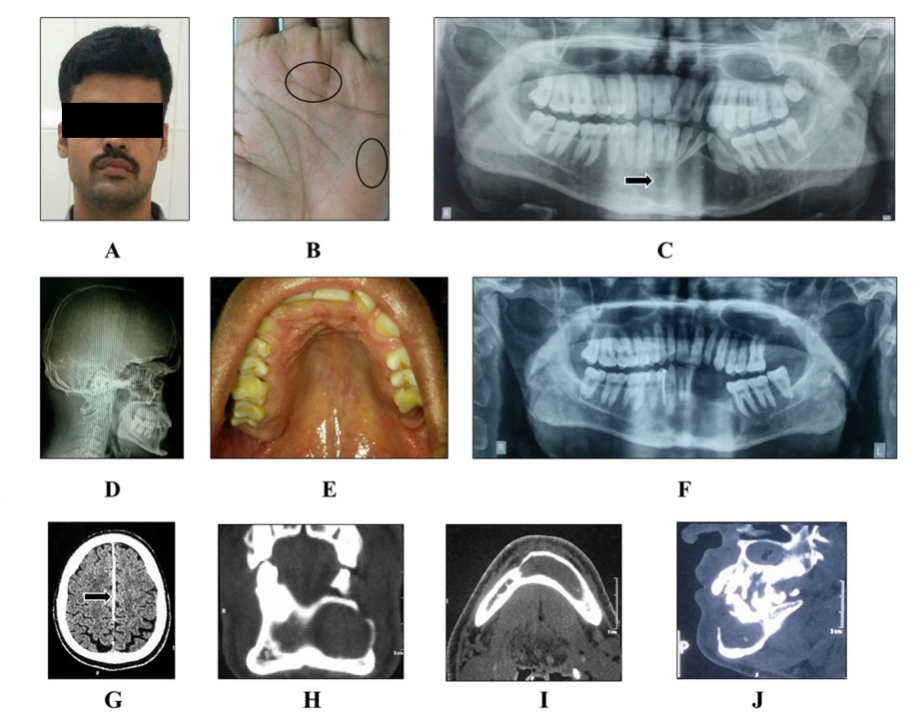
(A) Extraoral profile; (B) Palmar pits; (C) Panoramic radiograph; (D) Lateral cephalogram; (E) Intraoral examination, (F) Postoperative panoramic radiograph; (G, H, I, J) CT scan
